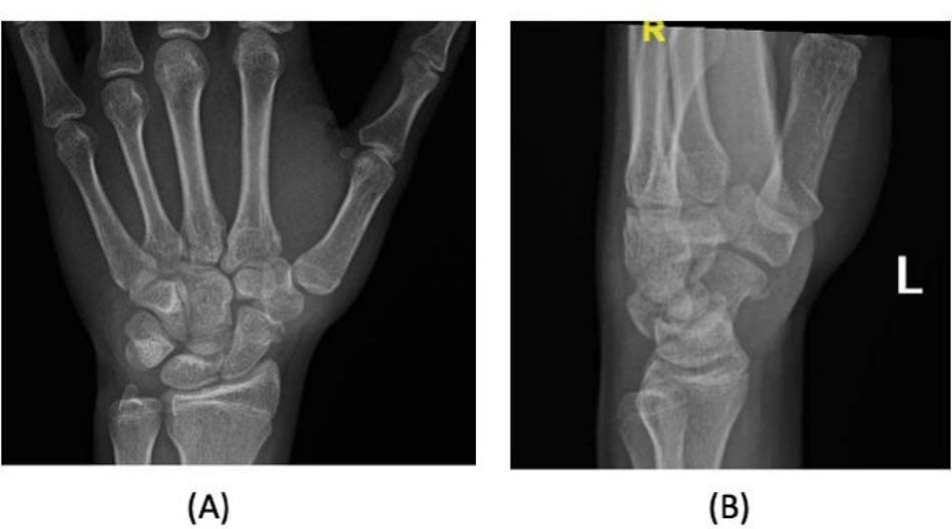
Initial injury posteroanterior and lateral radiograph demonstrating left wrist.
